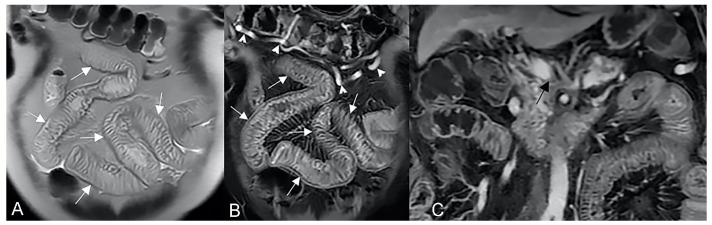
A case of small bowel ischemia in a 61-year-old male patient. (A) Coronal T2-weighted and (B,C) coronal T1-weighted after gadolinium injection images show diffuse thickening of small bowel loops (white arrows) with stratified contrast enhancement due to thrombosis of superior mesenteric vein (black arrow in (C)). Multiple collateral veins (arrowheads in (B)) and free abdominal fluid are also observed.
